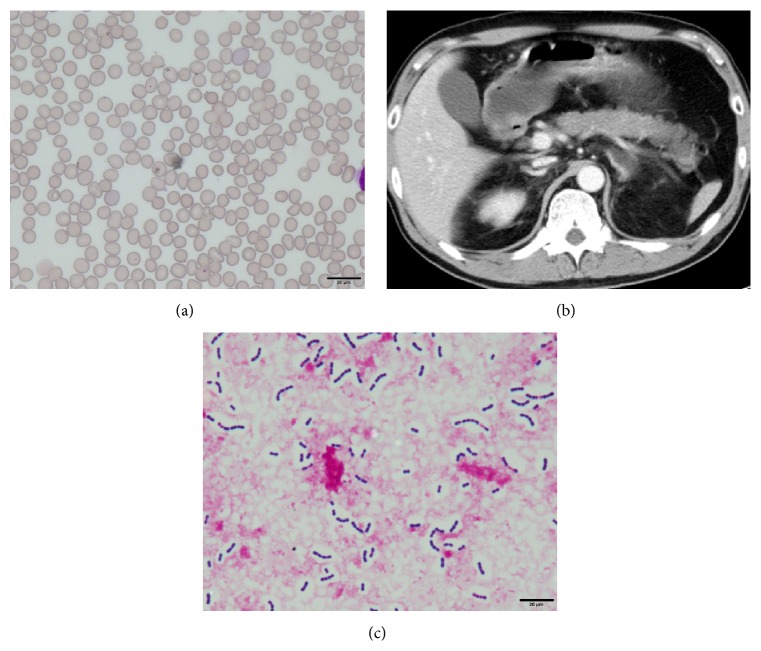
Peripheral blood smear, CT, and blood culture. (a) Peripheral blood smear revealed Howell-Jolly bodies. (b) Contrast CT demonstrated a hypoplastic spleen and retrospective fat tissue stranding around the left adrenal gland. (c) A blood culture revealed streptococci with capsules.
